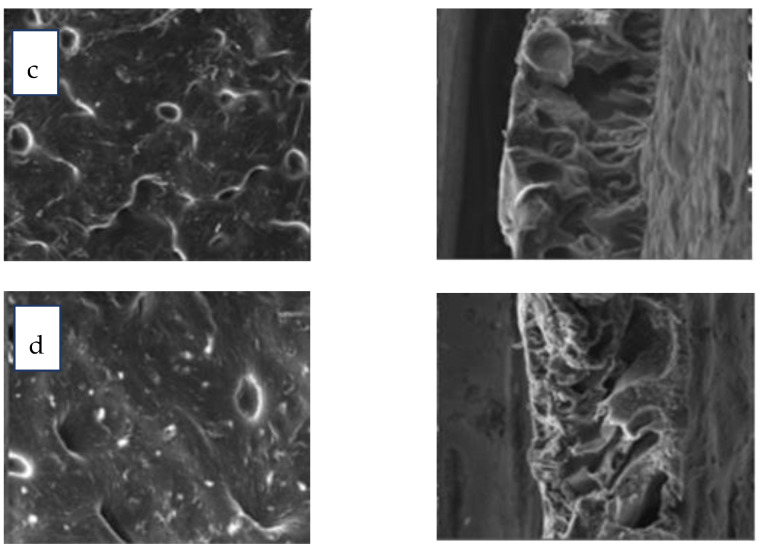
SEM images of the surface (magnification 800) and corresponding cross-section (magnification 2000) (a) Pure CTA, (b) 0.1 wt% wt% CA/GO, (c) 0.3 wt% wt% CA/GO, and (d) 0.5 wt% wt% CA/GO.
